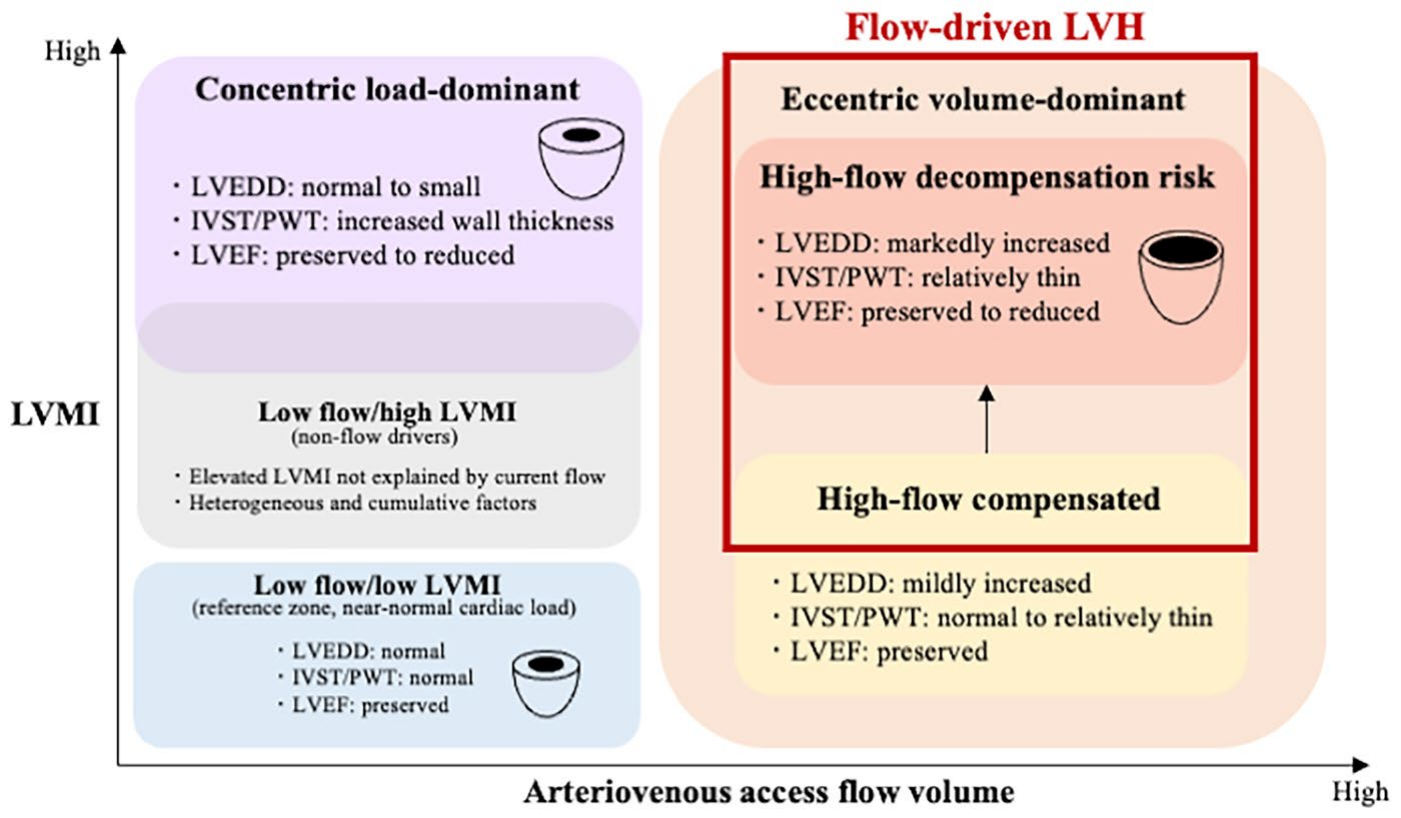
Flow–cardiac load phenotype map integrating arteriovenous access flow volume, the LVMI, and echocardiographic features. The framework primarily distinguishes between ‘eccentric volume-dominant’ and ‘concentric load-dominant’ remodeling patterns and provides a practical taxonomy for flow-driven left ventricular remodeling. LVMI, left ventricular mass index; LVEDD, left ventricular end-diastolic diameter; IVST, interventricular septal thickness; PWT, posterior wall thickness; LVEF, left ventricular ejection fraction; LVH, left ventricular hypertrophy.
